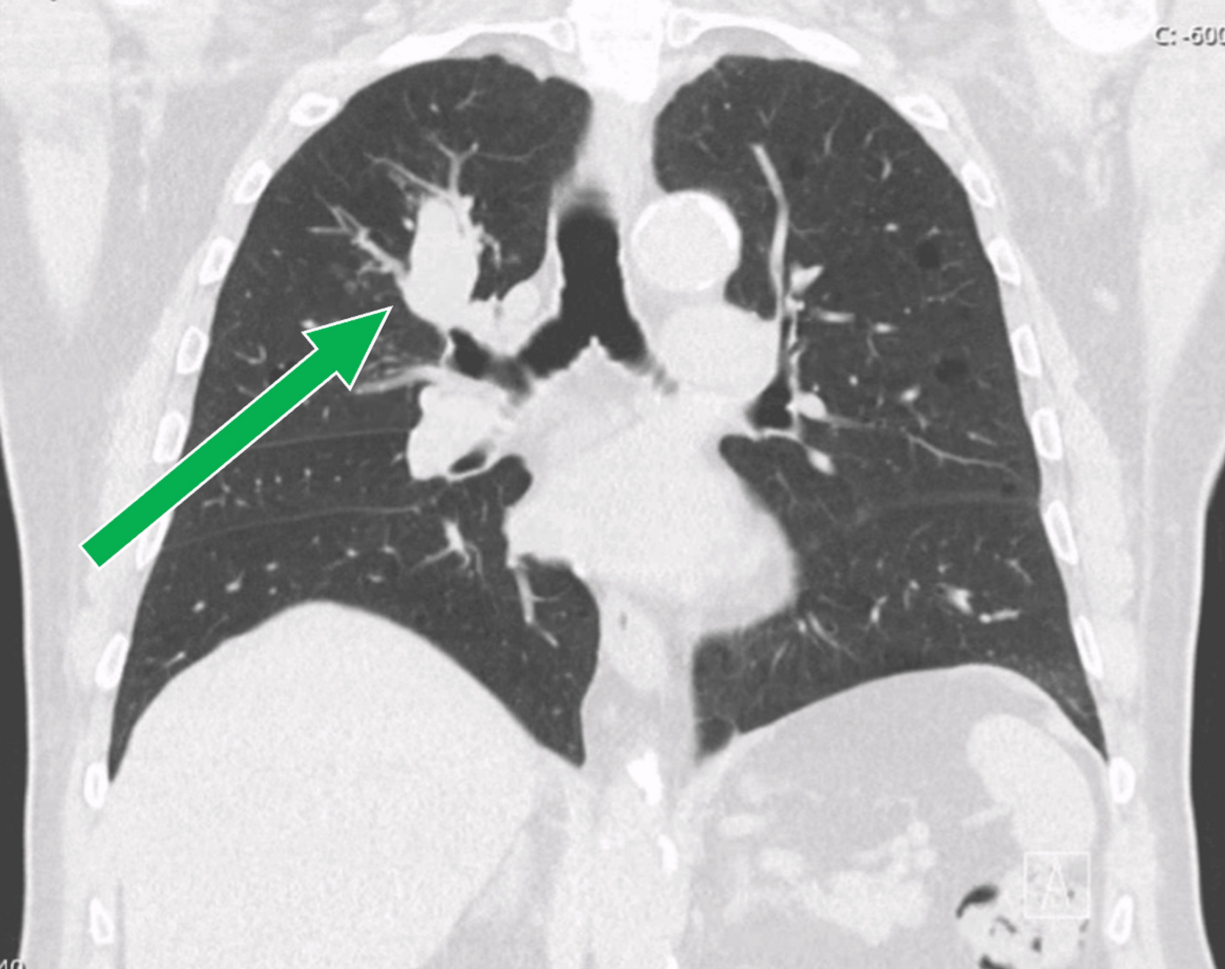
Chest CT - coronal plane. The green arrow indicates the right para-hilar lesion.
